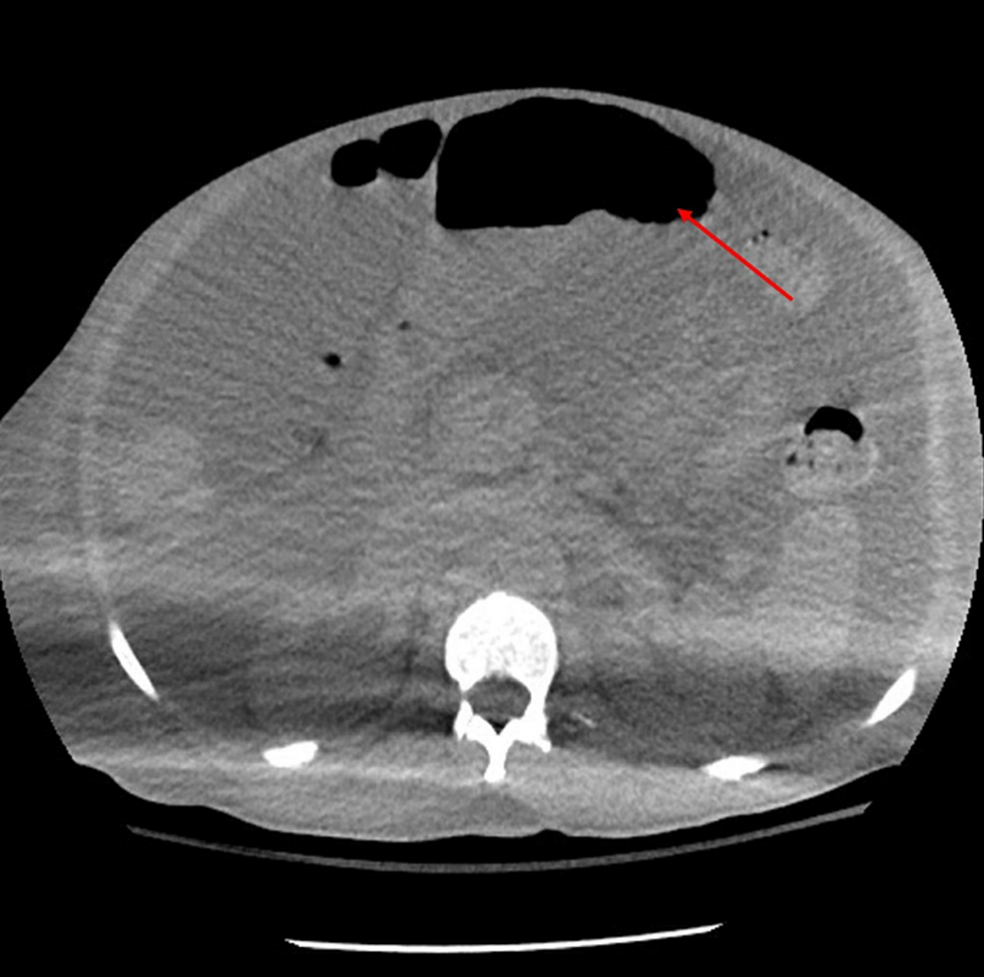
CT of the abdomen pelvis showed huge amount of ascites without evidence of intra-abdominal abscess. CT, computed tomography
